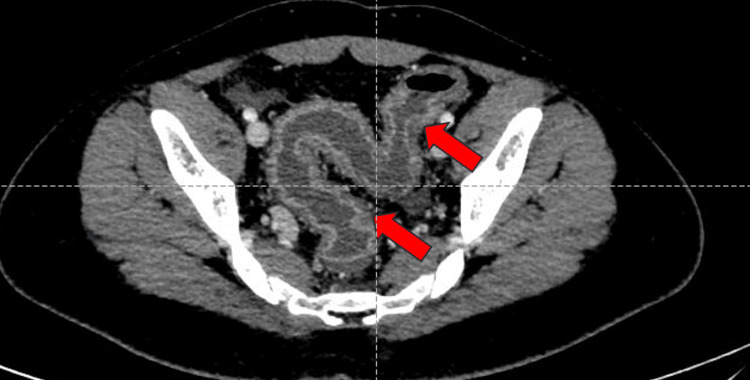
CECT-A/P axial section: diffuse wall thickening of the sigmoid colon and rectum with surrounding fat stranding and vessel engorgement (red arrows). CECT-A/P: contrast-enhanced computed tomography of the abdomen and pelvis
